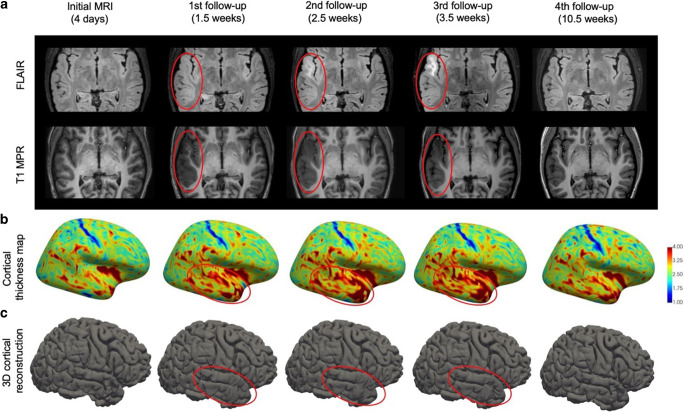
a Serial MRI scans obtained at baseline (4 days post-event) and at follow-up intervals at 1.5, 2.5, 3.5, and 10.5 weeks post-event. Cortical swelling in the right frontal and temporal operculum were observed at 1.5 weeks days post-event (first follow-up MRI), persisted through second and third follow-up at 2.5 and 3.5 weeks with concurrent subcortical edema and showed significant regression by 10.5 weeks. Progression and regression of cortical swelling can be seen in b cortical thickness maps and c 3D cortical surface reconstructions, which were generated by registering structural MRI data to the MNI152 space, cortical surface reconstruction in Vox2Cortex-Flow, manual revision for accuracy and visualization on an inflated FsAverage template using PyVista in Python
